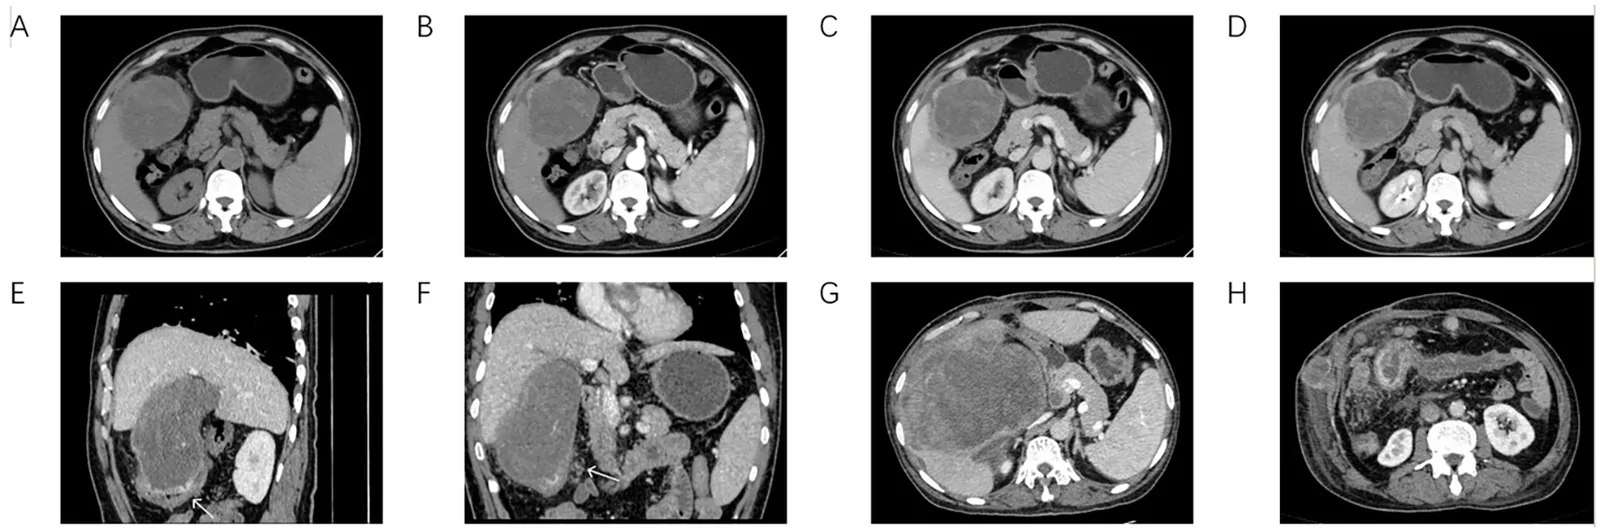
(A) Non-contrast CT scan showing an enlarged gallbladder with patchy soft tissue density shadows within; (B) Contrast-enhanced arterial phase; (C) Contrast-enhanced venous phase; (D) Contrast-enhanced delayed phase; (B–D) demonstrate patchy, progressive enhancement of the intra-gallbladder lesion; (E). Contrast-enhanced scan, venous phase, sagittal reconstruction: blurred fat planes at the arrow indicate tumour invasion into the hepatic flexure of the colon; (F). Contrast-enhanced scan, venous phase, coronal reconstruction: multiple small vessels clustered at the arrow; (G). Follow-up examination 2 months post-operatively: massive mass shadow visible in the gallbladder fossa; (H). Multiple soft tissue nodules noted in the right subcutaneous space of the lower abdomen and the right omentum.
